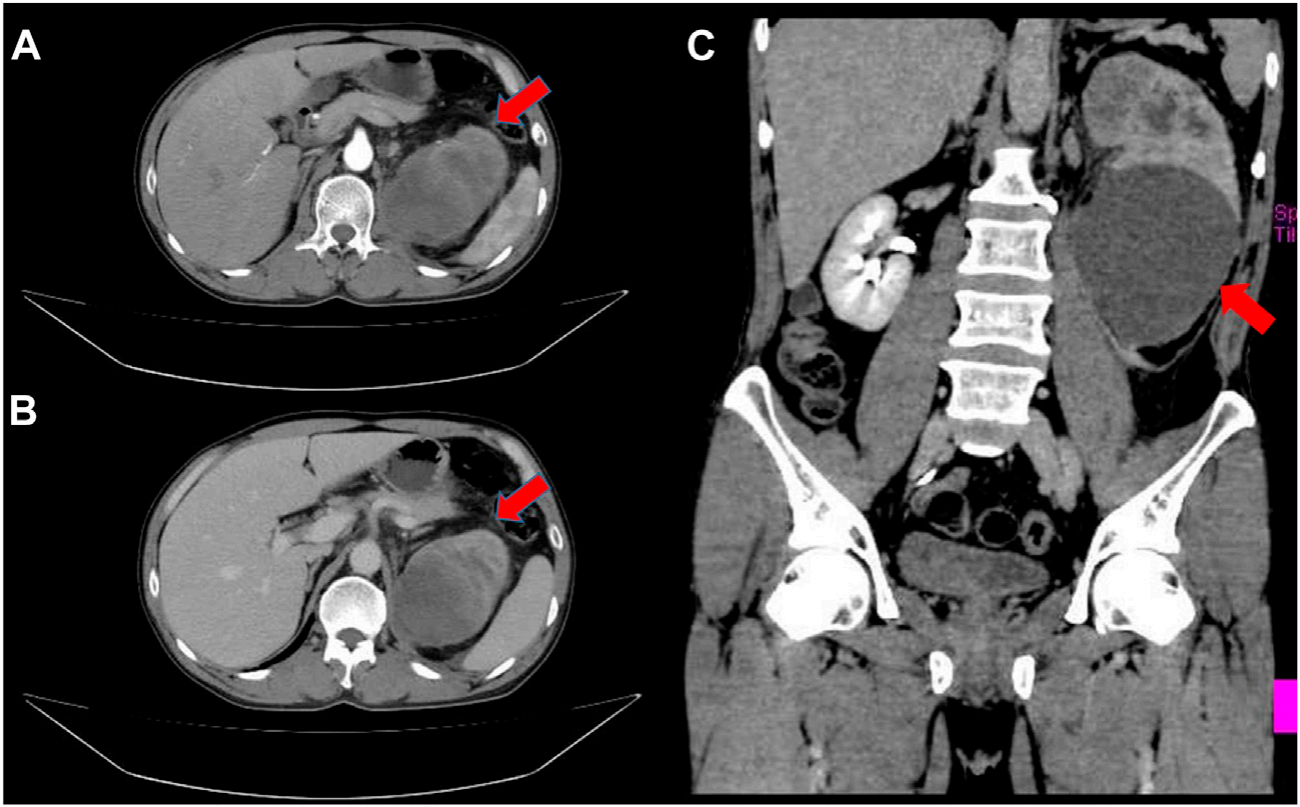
Enhanced Computed tomography revealed a large cystic solid tumor in the left kidney. (A) An arterial enhancement (red arrow); (B) A venous enhancement (red arrow); (C) Coronal plane (red arrow).
